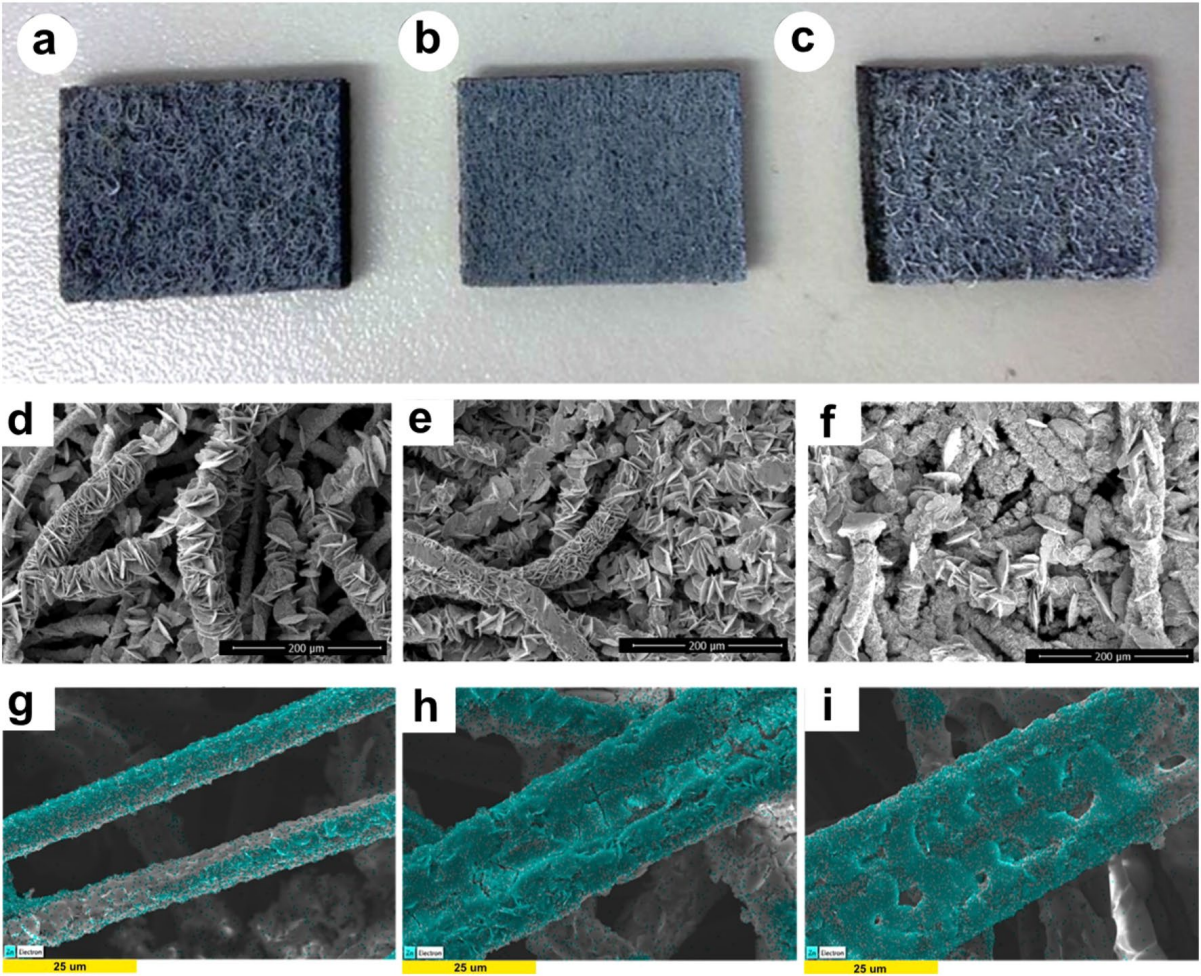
Photos of the as-prepared electrodes after zinc electrodeposition: (a) CF, (b) PVDF-CF and (c) G-PVDF-CF; FESEM images: (d) CF, (e) PVDF-CF and (f) G-PVDF-CF electrodes after electrodeposition of Zn; energy dispersive X-ray (EDX) mapping analysis of (g) CF, (h) PVDF-CF and (i) G-PVDF-CF electrodes after electrodeposition of Zn.
